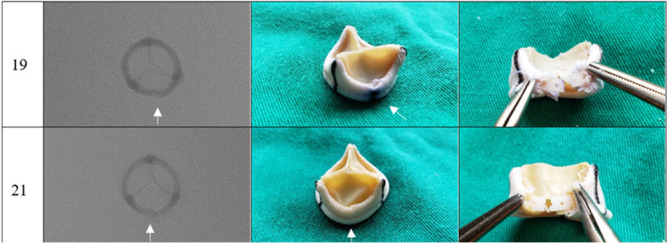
Cardioprótese Premium prosthesis after fracture, sizes 19mm and 21mm. Radiography (superior-inferior projection) and photographs (intact lining and dissected lining). Arrow corresponding to the fracture site.
